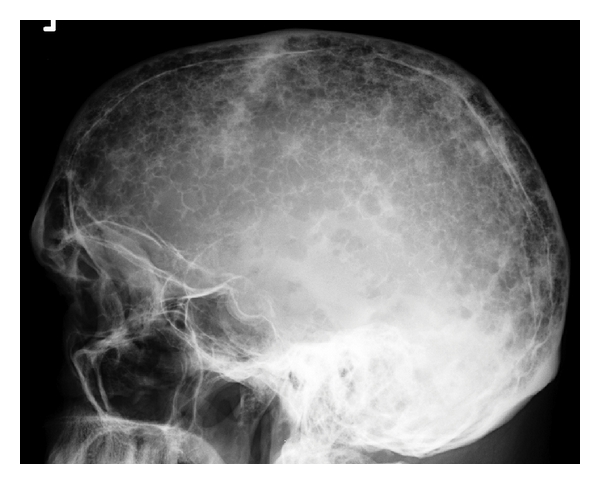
Lateral radiograph skull: diffuse lytic lesions giving classical “pepper pot skull” appearance.
